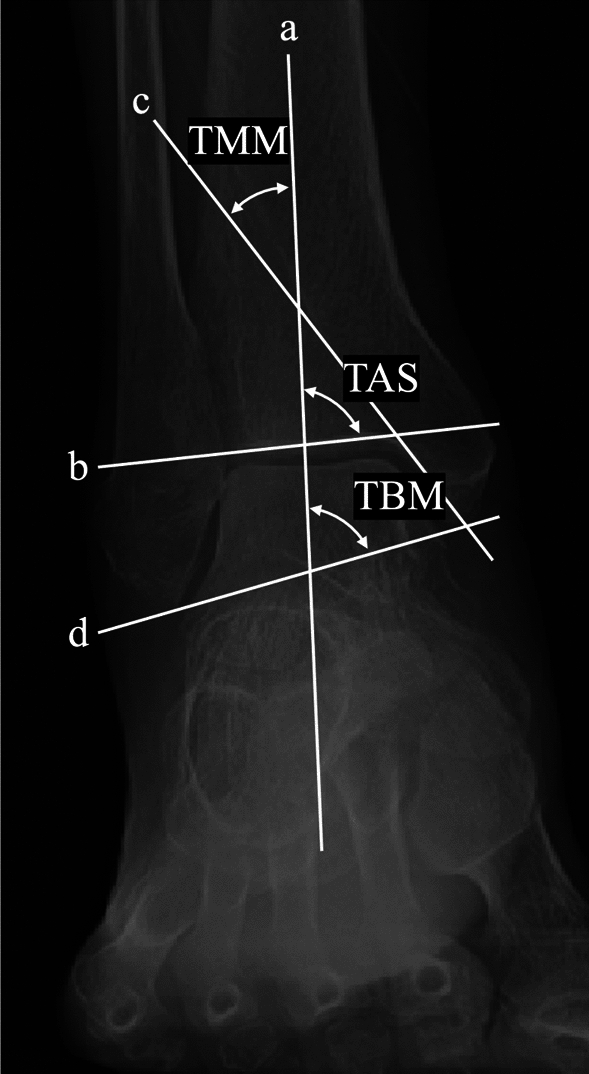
Radiographic measurements of morphometric parameters on whole-leg anteroposterior weight-bearing radiographs. Line (a) represents the mechanical axis of the tibia, (b) the subchondral plate of the distal tibial articular surface, (c) the subchondral plate of the medial malleolus articular surface, and (d) the line connecting the tips of both malleoli. The tibial anterior surface angle (TAS) is defined as the angle between lines (a) and (b), the tibial medial malleolus angle (TMM) is the angle between lines (a) and (c), and the tibial bimalleolus angle (TBM) is the angle between lines (a) and (d)
